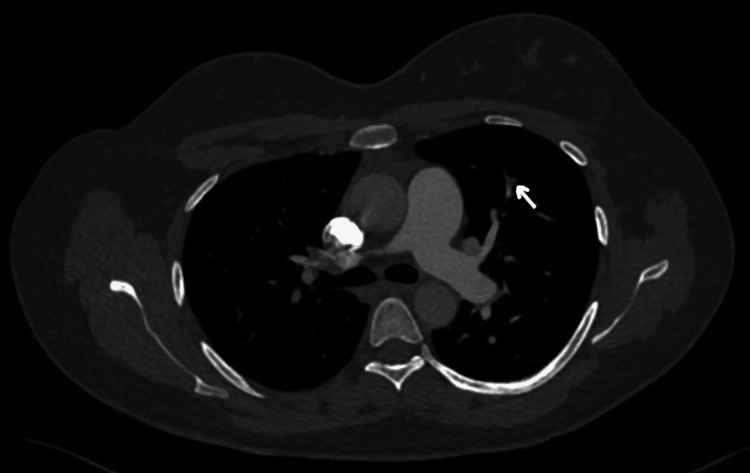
CT pulmonary angiogram (left segmental branches) There is evidence of filling defects seen in the segmental branches of the left lung
